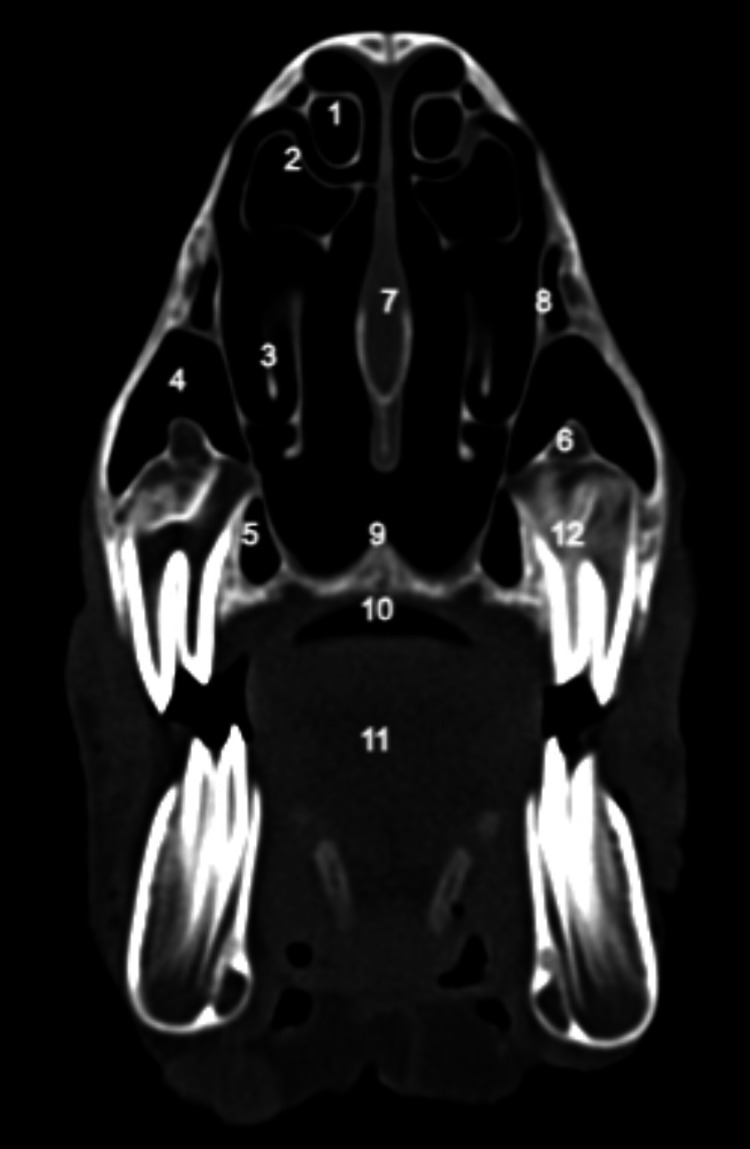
Coronal CT scan at the level of the second molar tooth 1: pneumatized superior turbinate; 2: pneumatized middle turbinate; 3: inferior turbinate with its superior component; 4: lateral chamber of maxillar sinus; 5: medial chamber of maxillar sinus; 6: infra: orbital canal; 7: nasal septum; 8: superior chamber of maxillar sinus; 9: the palatine process of maxilla; 10: hard palate; 11: body of the tongue; 12: second molar tooth.
